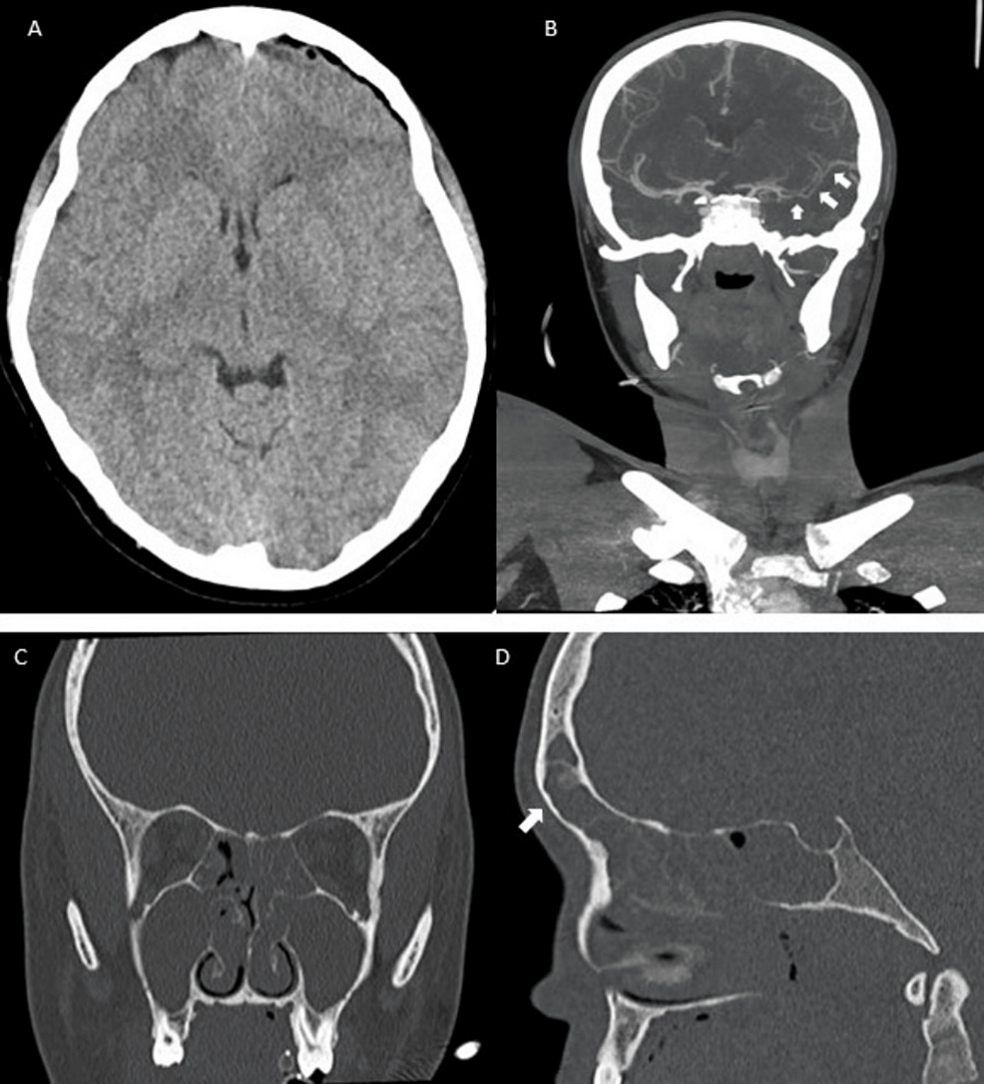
Cerebral computed tomography (CE-CT) angiography and maxillofacial CT at presentation. A - CE-CT imaging. The arrow indicates pneumocephalus; B - Tomography angiography. The arrow indicates reduction in middle cerebral artery segments caliber (M1, M2 and M3); C - Coronal plane maxillofacial CT demonstrates pansinusite; D – Sagittal plane maxillofacial CT. The arrow indicates the left frontal sinusitis.
